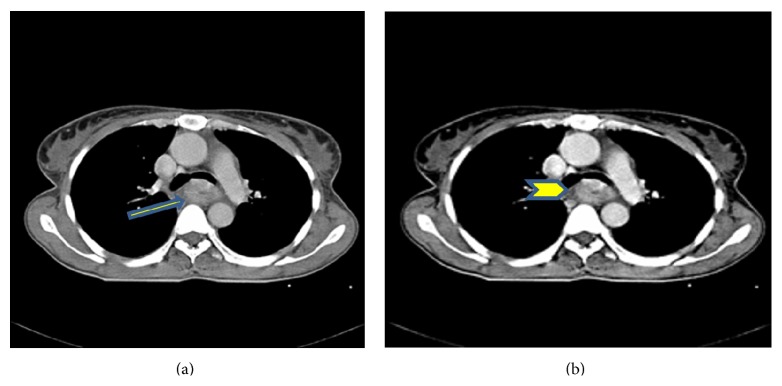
Computed tomography of esophageal mass (arrows) before neoadjuvant therapy.
